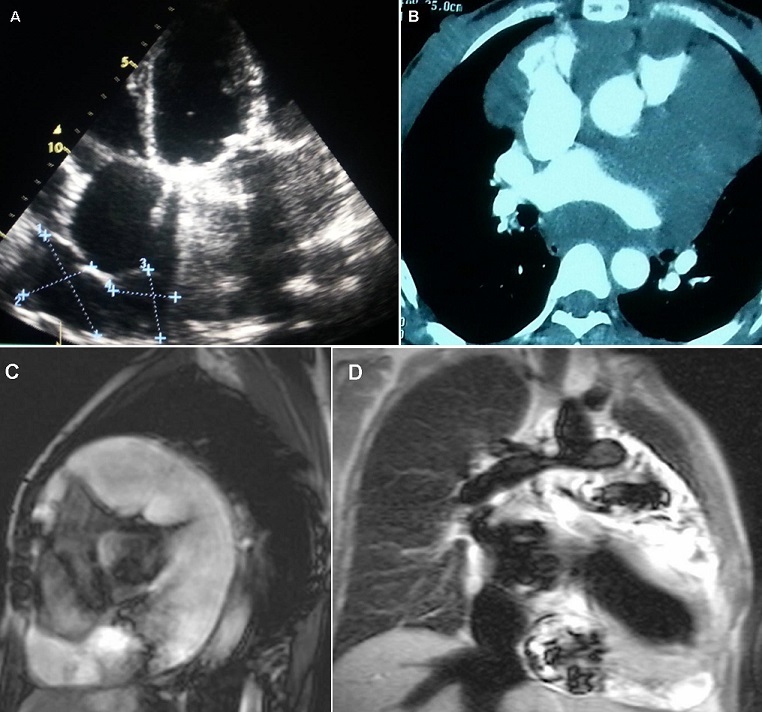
(A) axial Transthoracic echocardiography, Multiples cysts of pericardium, associated with signs of adiastole; (B) Axial contrast-enhanced CT scan of cheest. Abundant septated pericardial effusion, mullticystic extending into the mediastinum; (C) Heart MRI, T2-weighted black blood spin-echo images Pericardial constriction with numerous pericardial cysts, with intermediate signal intensity; (D) Heart MRI. T2-weighted STIR sequences Pericardial cysts, with high signal intensity
